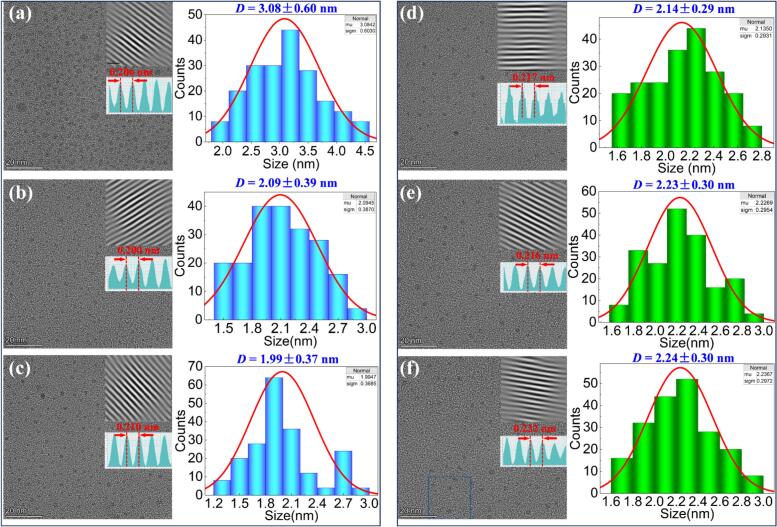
(a–c) TEM images and corresponding particle size distributions of CDs synthesized at flow velocities of (a) 0.2 mL/min, (b) 0.5 mL/min, and (c) 0.8 mL/min without ultrasonic treatment. Insets show HRTEM images and corresponding FFT patterns. (d–f) TEM images and particle size distributions of CDs synthesized at the same flow velocities, but with ultrasonic treatment.
